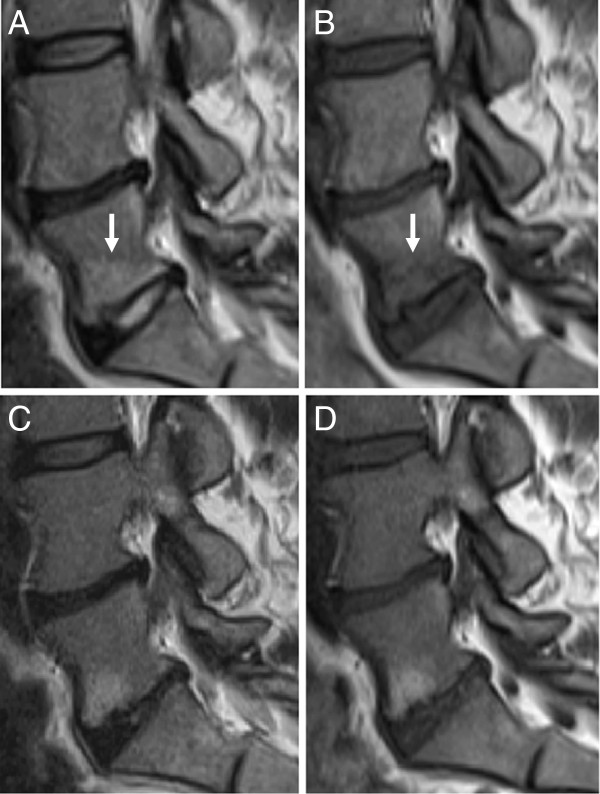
Altered type of Modic changes. Initial (A-B) and follow-up (C-D) sagittal magnetic resonance images of one patient. Type I Modic changes (arrows; high signal on T2-weigthed image A, low signal on T1-weighted image B) alters to type II (high signal on T2- and T1-weighted images C and D; images not shown revealed alteration to type II/I).
